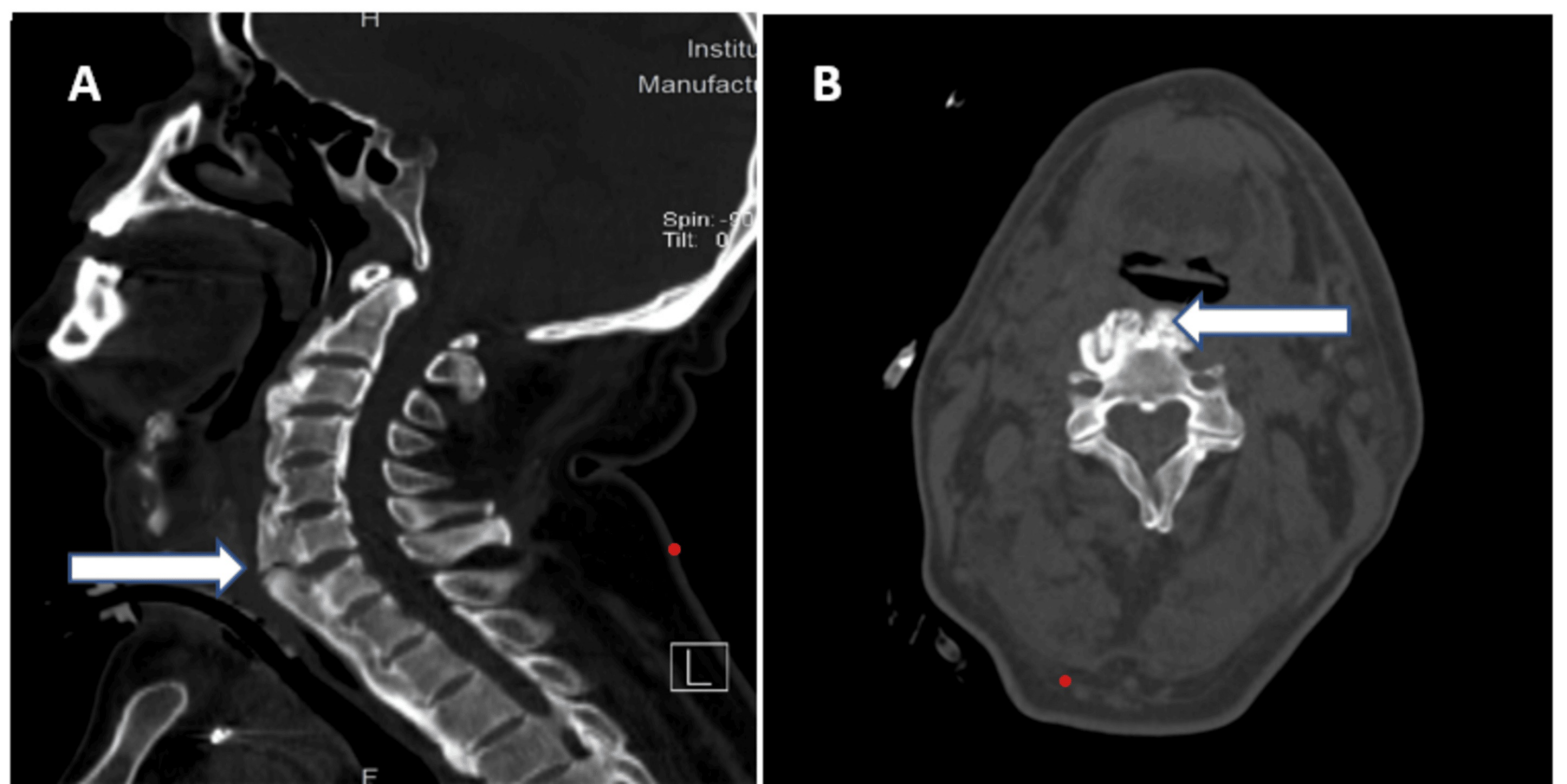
Sagittal (A) and axial (B) non-contrast CT scans in bone window of the cervical spine. White arrow shows exuberant and bulging anterior-bridging osteophytes causing indentation of the prevertebral space.
